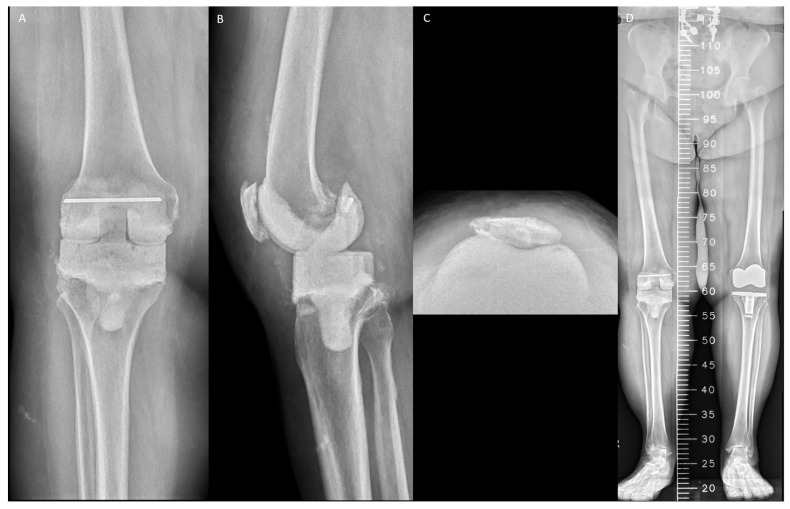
Radiographs of the articulating spacer obtained 1 week after resection arthroplasty: (A) standing anteroposterior view; (B) standing lateral view; (C) merchant view; and (D) scanogram of the lower limb.
